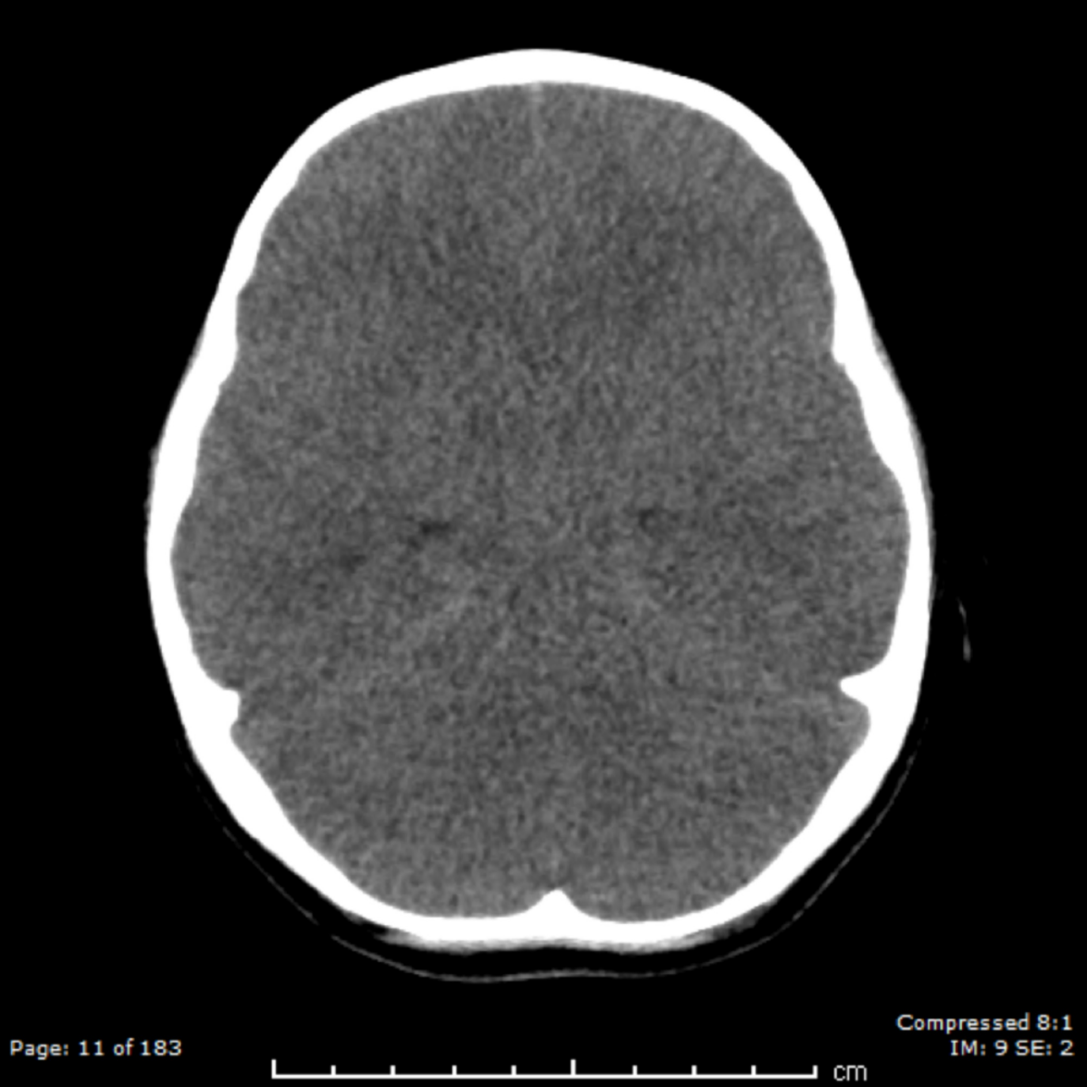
Head CT showing global cerebral edema
